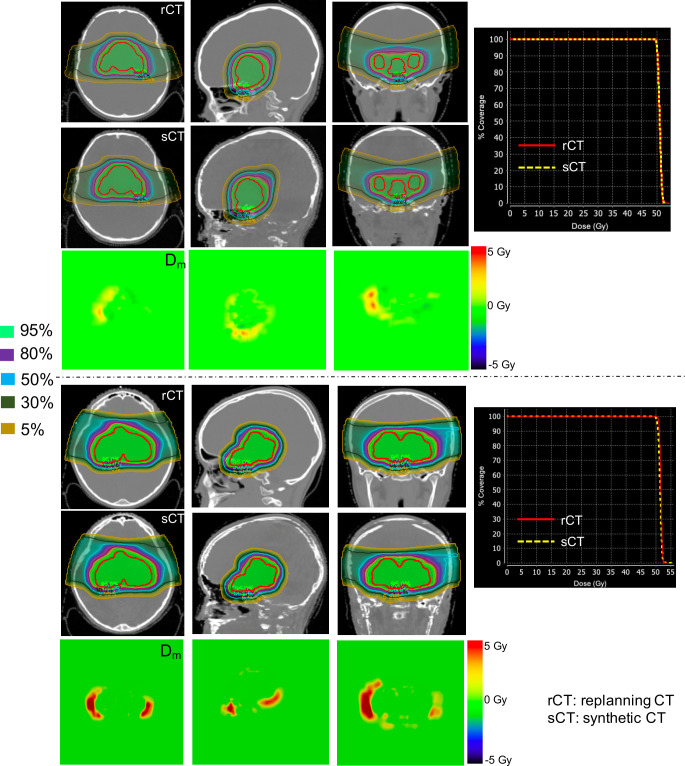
Differences in dose map and CTV DVH between sCT and replanning CT for the adapted plan for patient 5 (top panel) and patient 7 (bottom panel). Red contour = CTV. The dose map was generated for the total prescribed dose. Abbreviations: CT, computed tomography; CTV, CT cerebral venography; DVH, dose-volume histogram; sCT, synthetic computed tomography.
